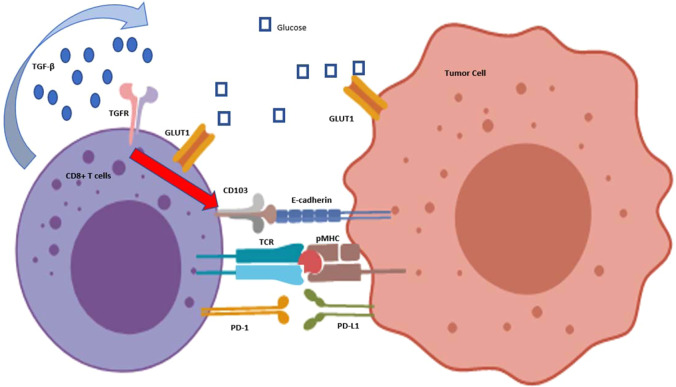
Characteristics of CD103 +  CTA-specific CTLs. Patient circulation-derived CTA-specific CD103+ CTLs exhibit self-production of TGF-β1 in its active form, which is potentially recognized by self-TGFR, mediating the self-maintenance of CD103 expression. This unique T-cell population also has elevated glucose consumption over time, and mediates faster recognition and killing of individual cancer cells. The presence and interaction between CD103 and its ligand E-cadherin on cancer cells would support the faster recognition. However, the presence or absence of PD-1 and its interaction with PD-L1 on cancer cells might influence the antitumor T-cell responses
